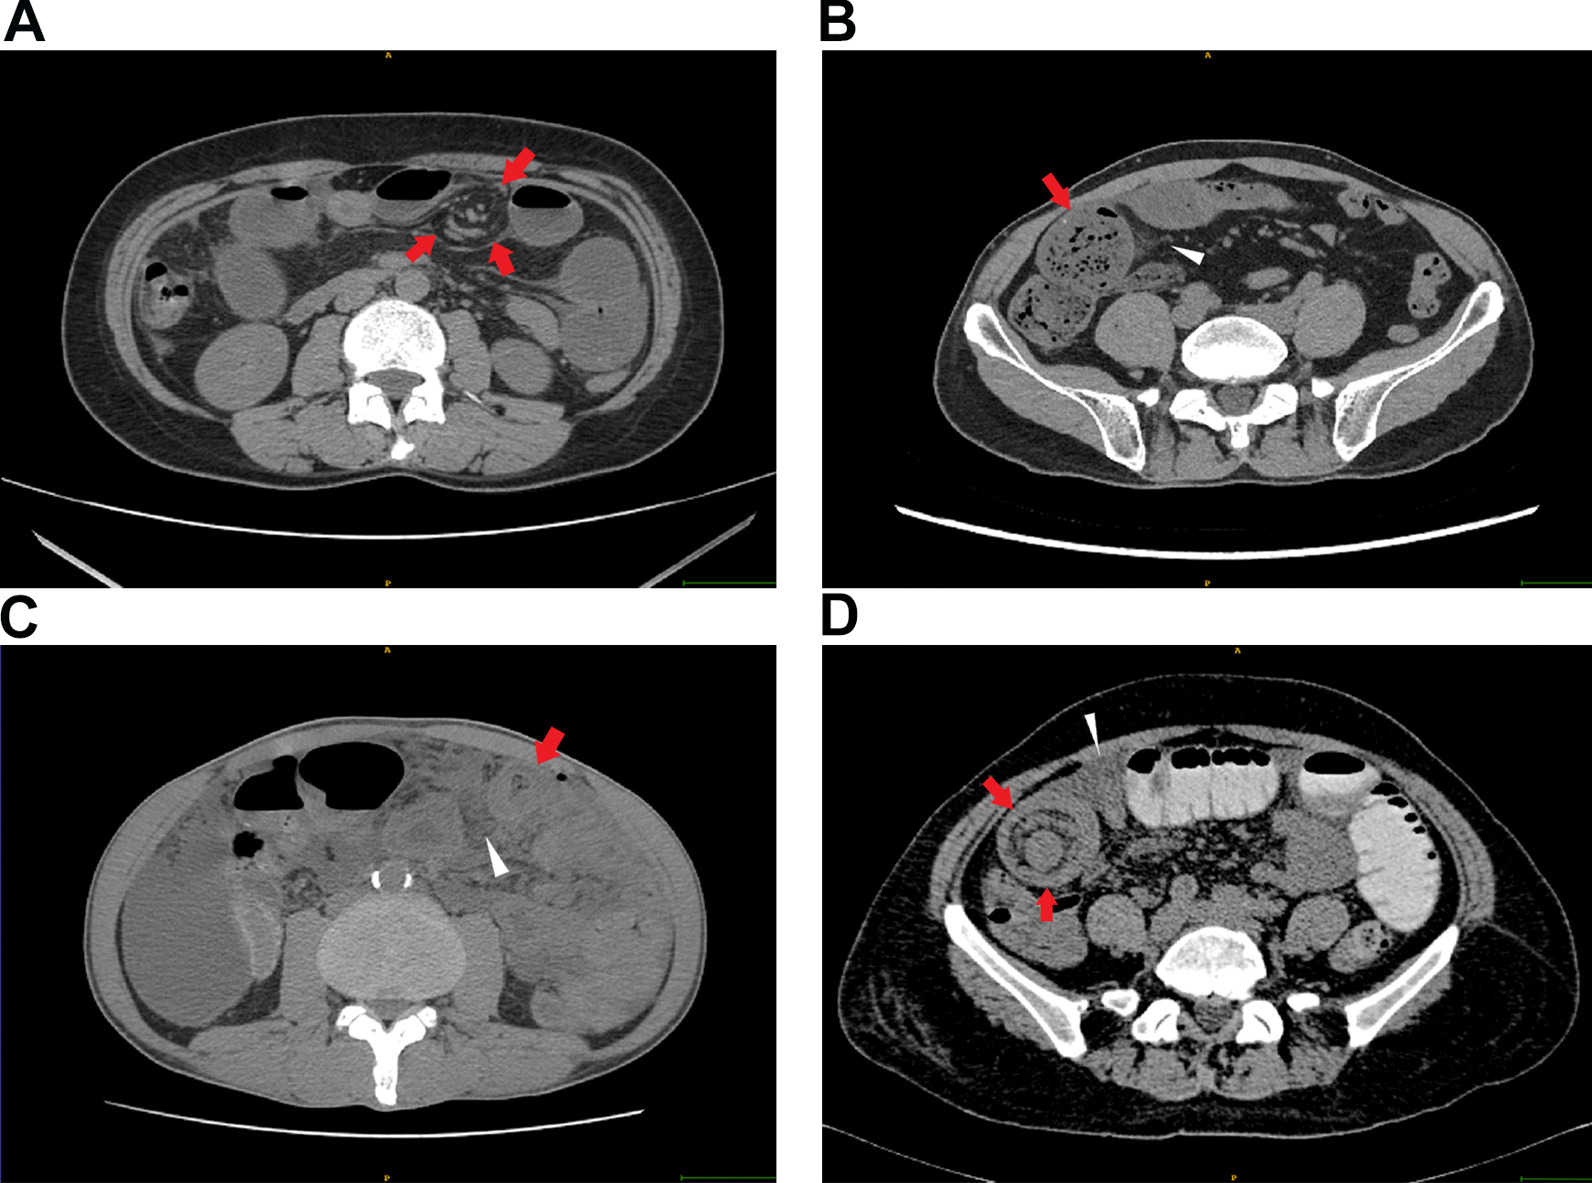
Images of CT findings. A a 49-year-old woman with adhesive small bowel obstruction. Axial CT of the abdomen confirmed the spiral sign of small bowel (red arrow). B a 51-year-old man with adhesive small bowel obstruction. Axial CT of the pelvis confirmed the small bowel feces sign(red arrow) and the mesenteric fluid (white triangle). C a 51-year-old man with inflammatory small bowel obstruction. Dilated, thickened loops of small bowel (red arrow) and mesenteric fluid (white triangle) could be observed. D a 70-year-old woman with intussusception. Axial CT images showed the concentric circle sign of small bowel (red arrow) and ascites (white triangle)
